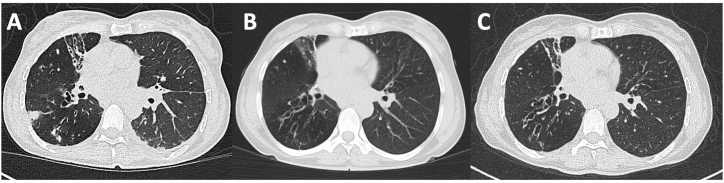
Axial thoracic CT images demonstrating bronchiectasis, mucus plugging, and extensive multifocal pulmonary consolidations (Fig. 1A). Follow-up CT scans after 8 weeks (B) and 12 weeks (C) of antibiotic therapy show bilateral regression of consolidations.
